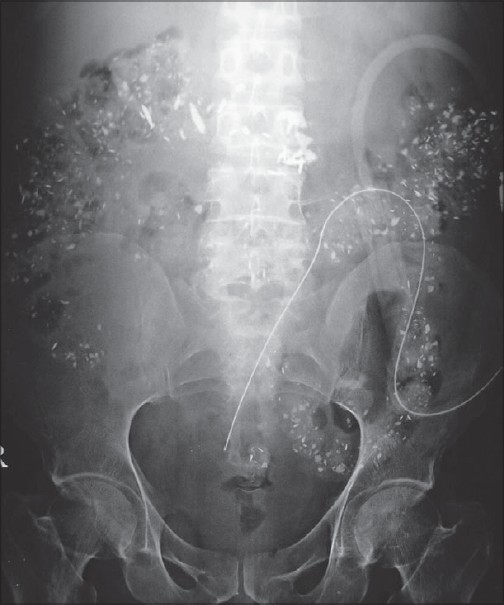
Plain X-ray abdomen (erect) of the patient
